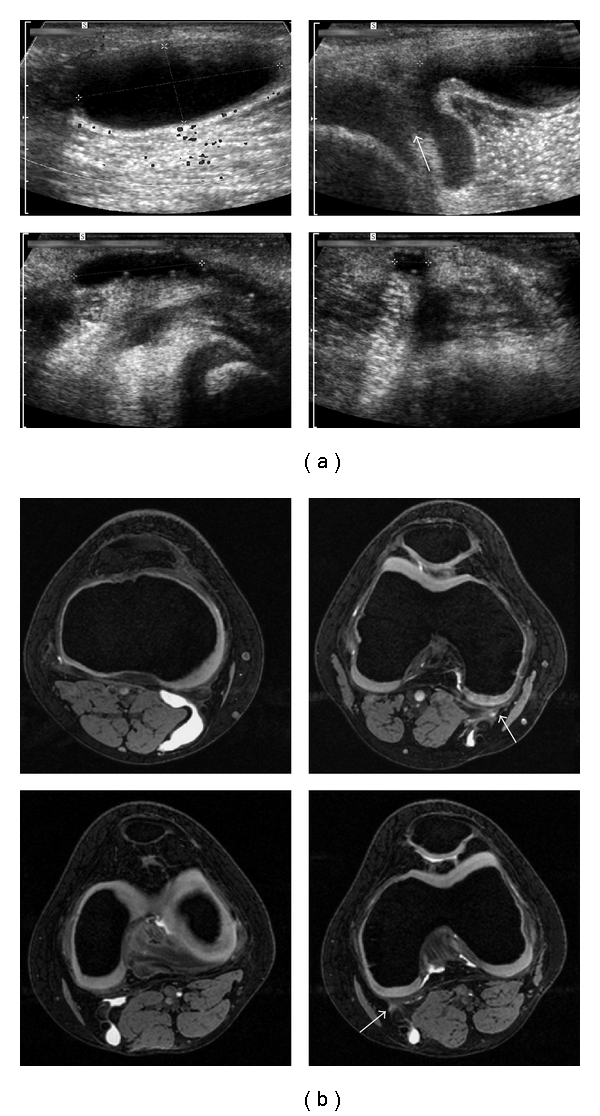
Bilateral Baker cysts in an 11-year-old boy diagnosed with joint hypermobility syndrome. (a) Ultrasonography showed anechoic cysts with a volume of 5.2 mL (right knee, upper row) and 2 mL (left knee, lower row). Communication with the joint space on the right side was described in the ultrasound report, though the documented image of the assumed site of communication (arrow) is not completely conclusive. Patient information blurred. (b) Magnetic resonance imaging in the same patient (3 Tesla MAGNETOM Trio, Siemens, transversal DESS sequence) proved Baker cysts of the right (upper row) and the left (lower row) popliteal fossa in the absence of other pathologies. Both cysts extend towards the joint space, exhibit fluid signal in the vicinity of the articular capsule and were assessed as communicating cysts.
